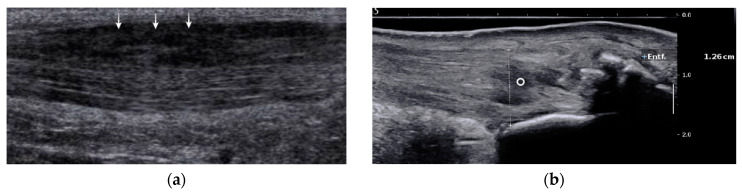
US images of partial Achilles tendon ruptures: (a) partial rupture in the dorsal part of the Achilles tendon midportion (arrows); (b) partial rupture in insertional area of the Achilles tendon (circle). US; ultrasound.
